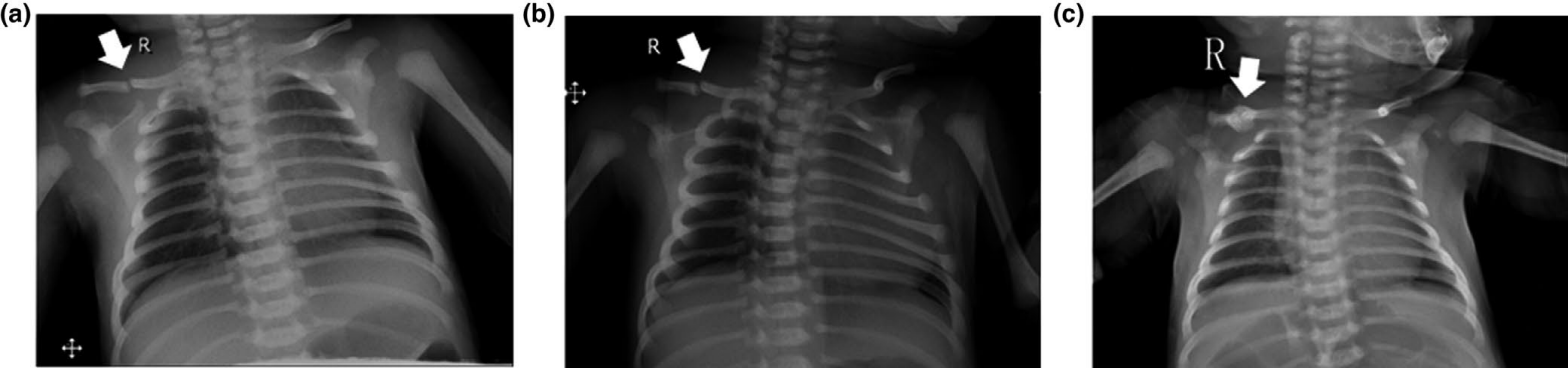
Radiographic features of the patient. Chest X‐rays at (a) 2 days of age; (b) 9 days of age; and (c) 30 days of age
